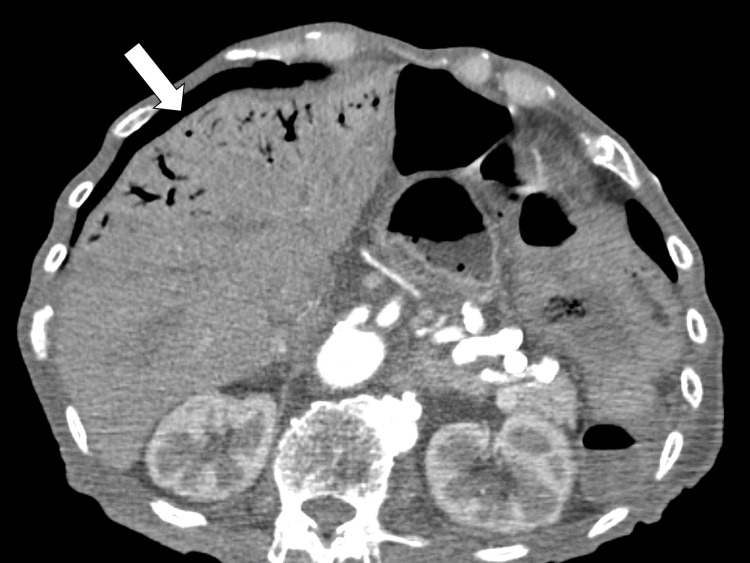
Contrast CT scan on the 17th day The CT revealed portal vein gas in the liver. CT, computed tomography
